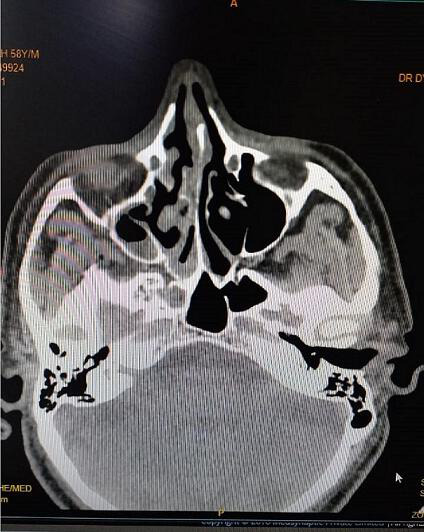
Case 1. CT PNS: the ethmoidal and maxillary nasal sinuses display an ill-defined heterogeneous soft tissue signal intensity of mucosal thickening.
